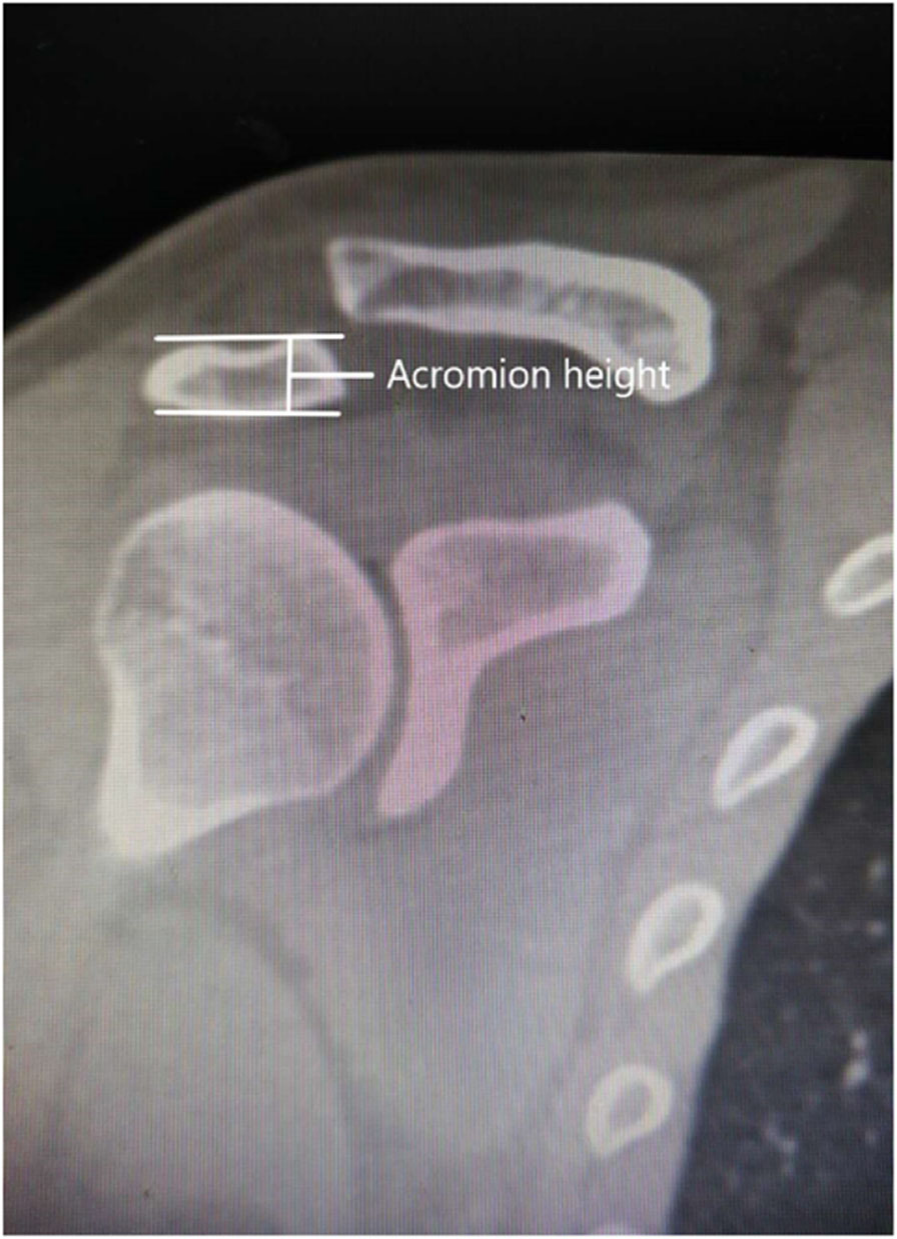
Measurement of the acromial height on coronal plane CT
